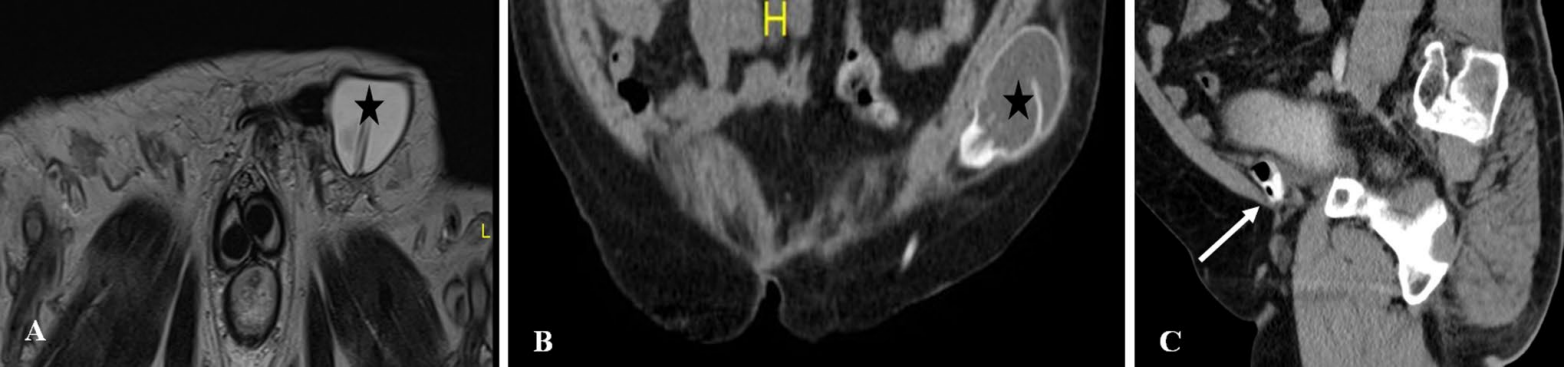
Complications involving reservoir. a Axial T2-weighted MRI shows the inguinal migration of pelvic reservoir (asterisk). b Coronal CT shows the abdominal wall placement of reservoir (asterisk) instead of usual pelvic placement. c Sagittal CT shows the collapsed contrast filled reservoir with air indicating reservoir failure (arrow)
